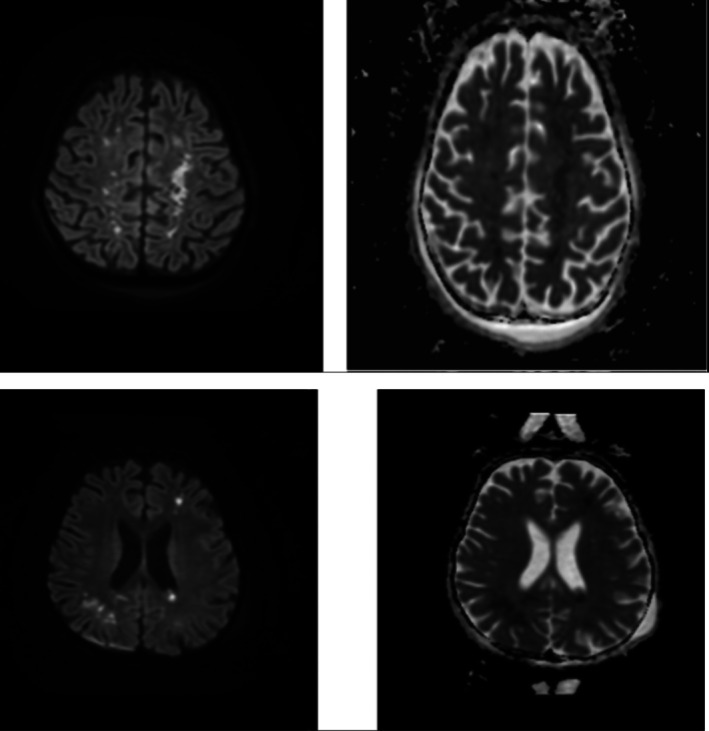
MRI brain showed diffusion weighted images and ADC map show multiple focal areas of diffusion restriction in bilateral centrum semiovale and subcortical white of frontal and parietal lobes bilaterally.
